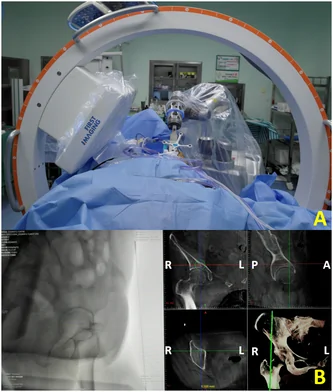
Preoperative bony structure image reconstruction. (A) The patient was placed in the supine position, and 3‐dimensional (3D) computed tomography scan of the affected right hip. (B) Reconstructed images of the affected right hip (oblique coronal view, oblique sagittal view, axial view, and 3D reconstruction, respectively).
